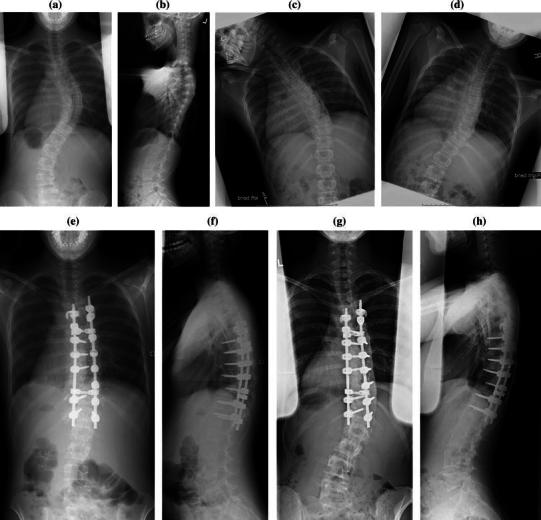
A 12 years and 8 months old, Risser grade 0, pre-menarchal female with Lenke type 1B idiopathic scoliosis. Pre-operative posterior-anterior (PA) (a), lateral (b), *left* bend (c), and *right* bend (d) radiographs demonstrate a 57° main thoracic curve. First-erect post-operative PA (e) and lateral (f), and 5-year post-operative PA (g) and lateral (h) radiographs demonstrate correction of the coronal plane deformity down to 15° with a T5–T12 posterior instrumented spinal fusion. The patient was fused to one level short of the stable vertebra. The deformity then progressed to 41° at final follow-up. At the most recent follow-up, the patient has developed a 16° thoracic rib prominence with worsening trunk shift to the right and waist asymmetry
